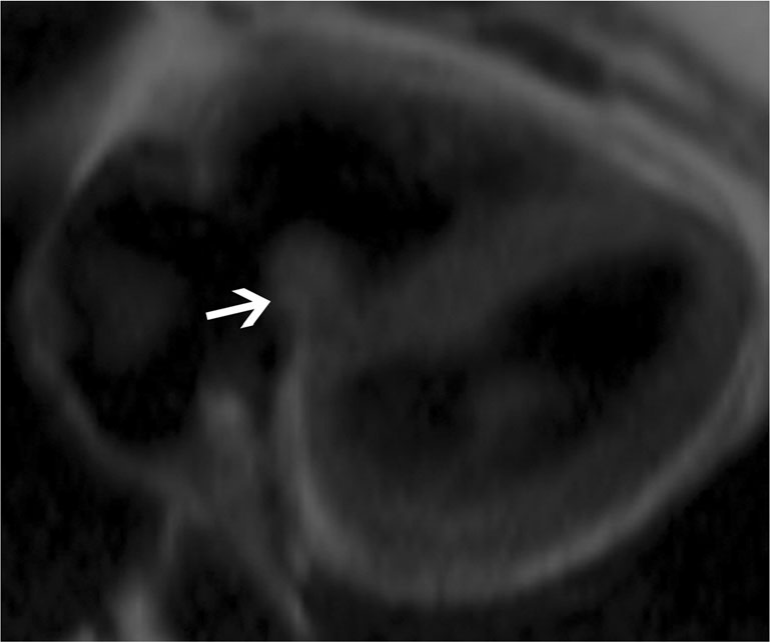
Lymphoma. Axial black-blood T2-weighted image shows a focal mass in the septal leaflet of the tricuspid valve in a patient with B-cell lymphoma
